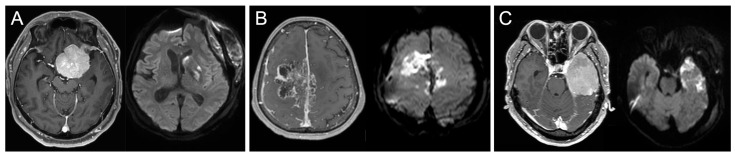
Postoperative DWI signal changes. Paired preoperative T1Gd and postoperative DWI are shown to demonstrate different patterns of postoperative DWI signal changes, including arterial (A) or venous (B) infarction or contusion (C).
